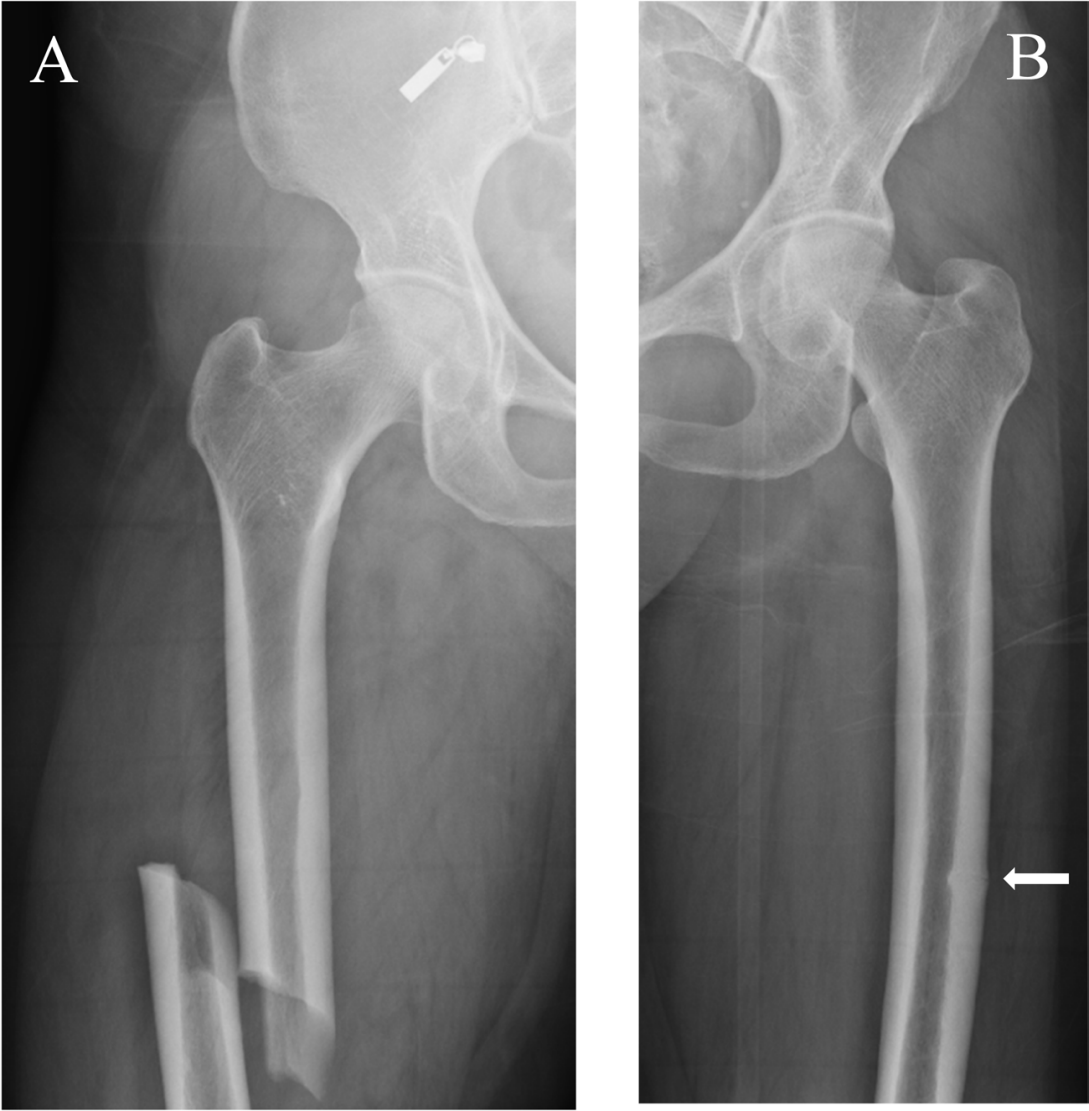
A 58-year-old woman with osteoporosis who presented with bilateral AFFs. (A) Anteroposterior radiograph of the right femur shows a complete AFF. (B) Anteroposterior radiograph of the left femur shows an incomplete AFF (white arrow)
